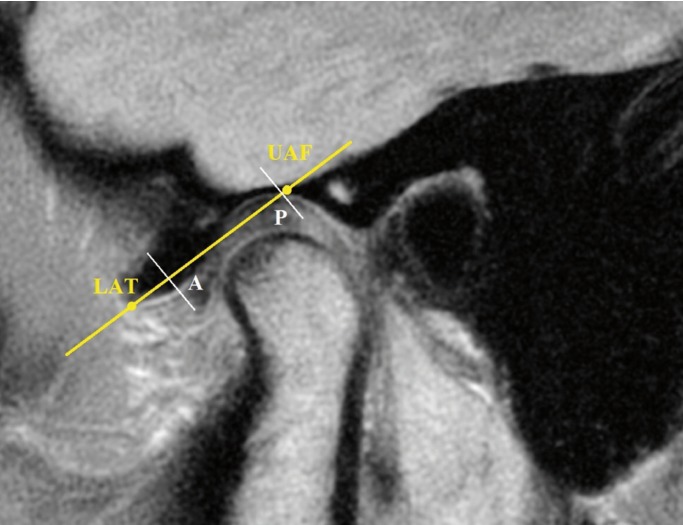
Evaluation of disc position on MR images.
